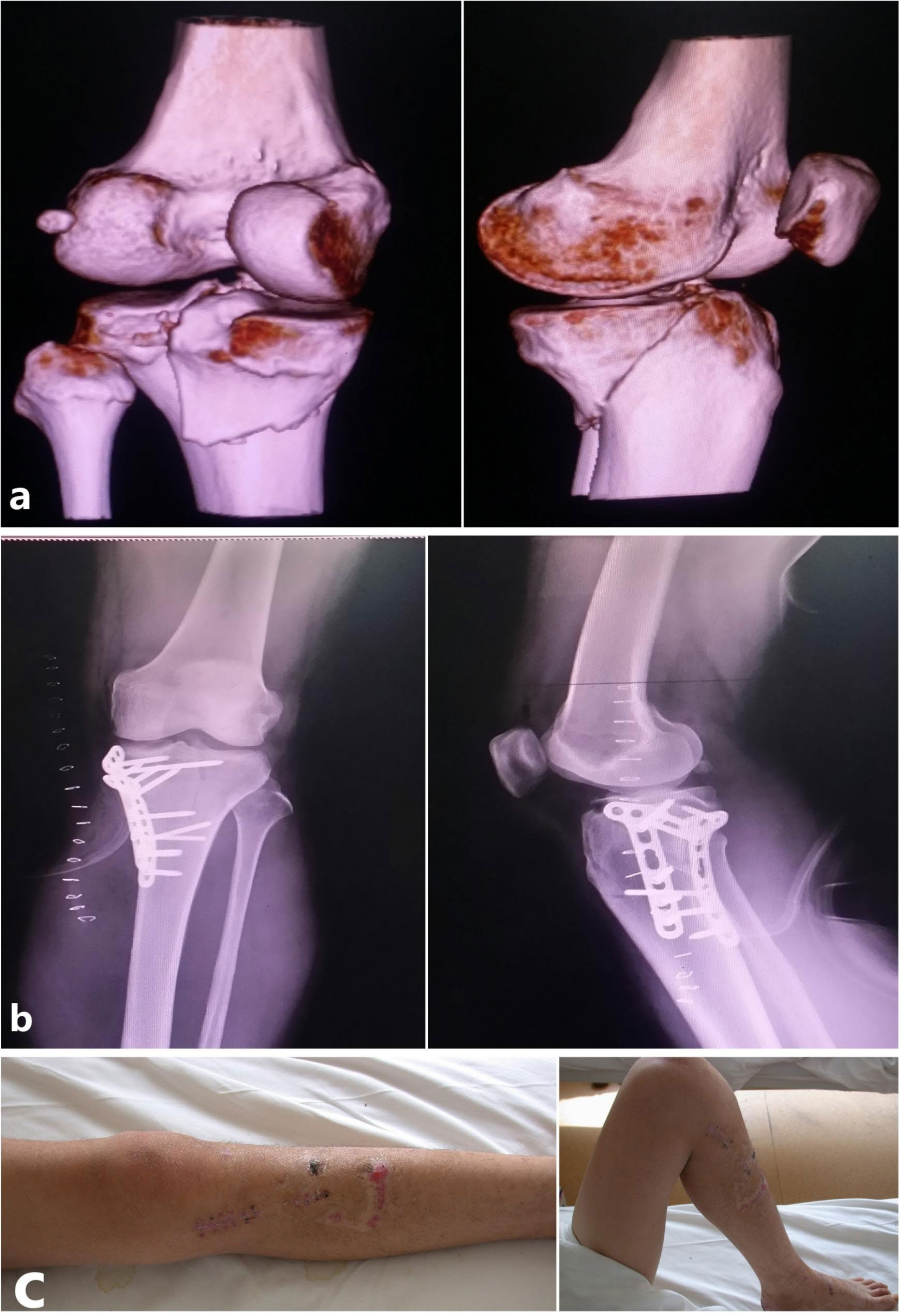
A typical case of a 22-year-old female with Schatzker IV tibial plateau fractures (flexion-varus-internal rotation type). (a) The features of this type were shown in CT images. (b) After the reduction of MFA, a plate should be placed upon MFA to fix the posteromedial fragment. Another plate was needed to strengthen the fixation of the medial condyle fracture. (c) 2 weeks postoperatively, the function of the knee was excellent
